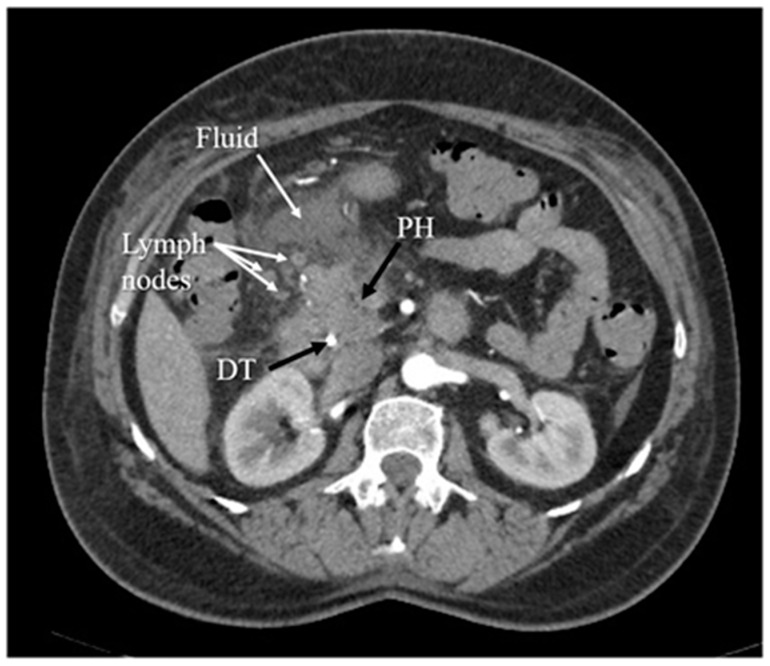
Inflammation of the head of the pancreas, with surrounding fluid and several enlarged lymph nodes. The arterial phase of CT. PH—head of the pancreas, DT—duodenal tube.
